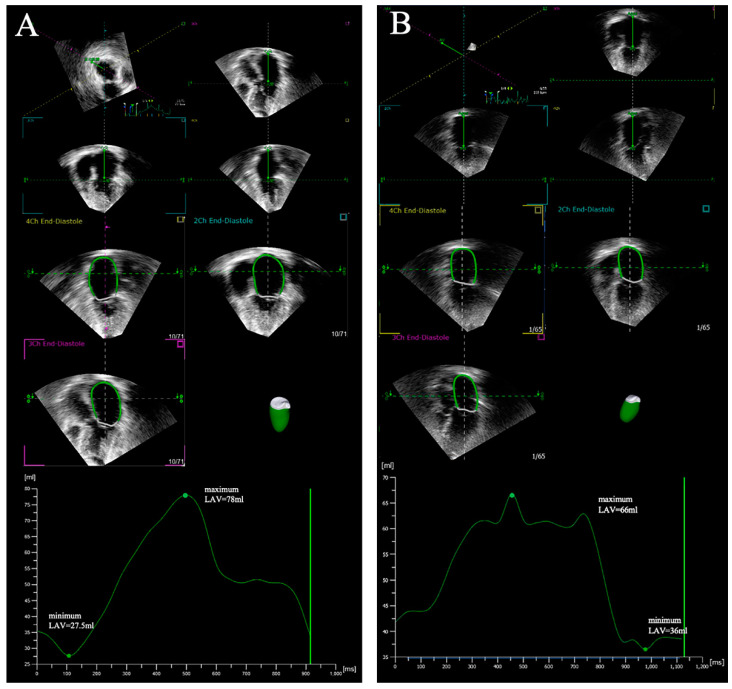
Representative measurements of LAV by three-dimensional echocardiography. Standard apical four-chamber view images of 20 consecutive cardiac cycles were stored, and LAV was measured in one of the cardiac cycles. (A) Reference points setting, LA endocardial border tracking and acquisition of 3D LAV curve in a subject with sinus rhythm; (B) Reference points setting, LA endocardial border tracking and acquisition of 3D LAV curve in a subject with AF.
